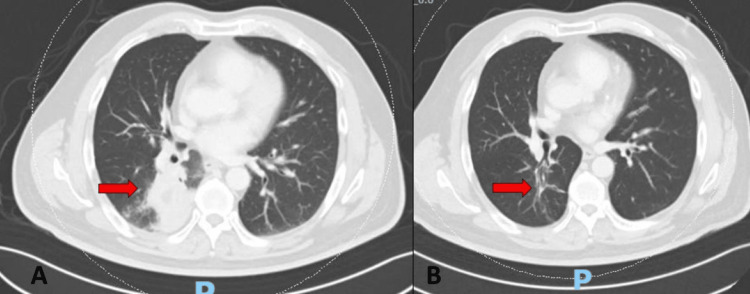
Computed tomography of the thorax view of the same location before (A) and after (B) corticosteroid treatment.
